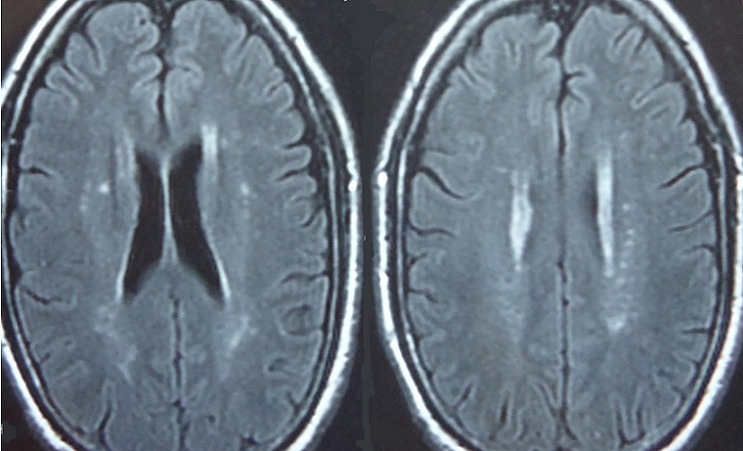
Flair weighted MRI sequences of two patients showing atypical presentations of neurobehçet disease
